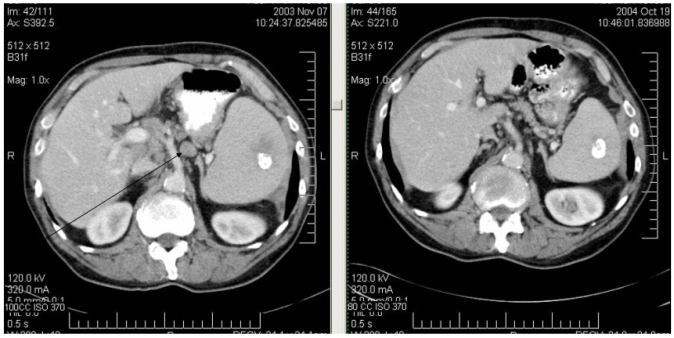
Patient 2: computed tomography scans showing decreased adenopathy and splenomegaly.
